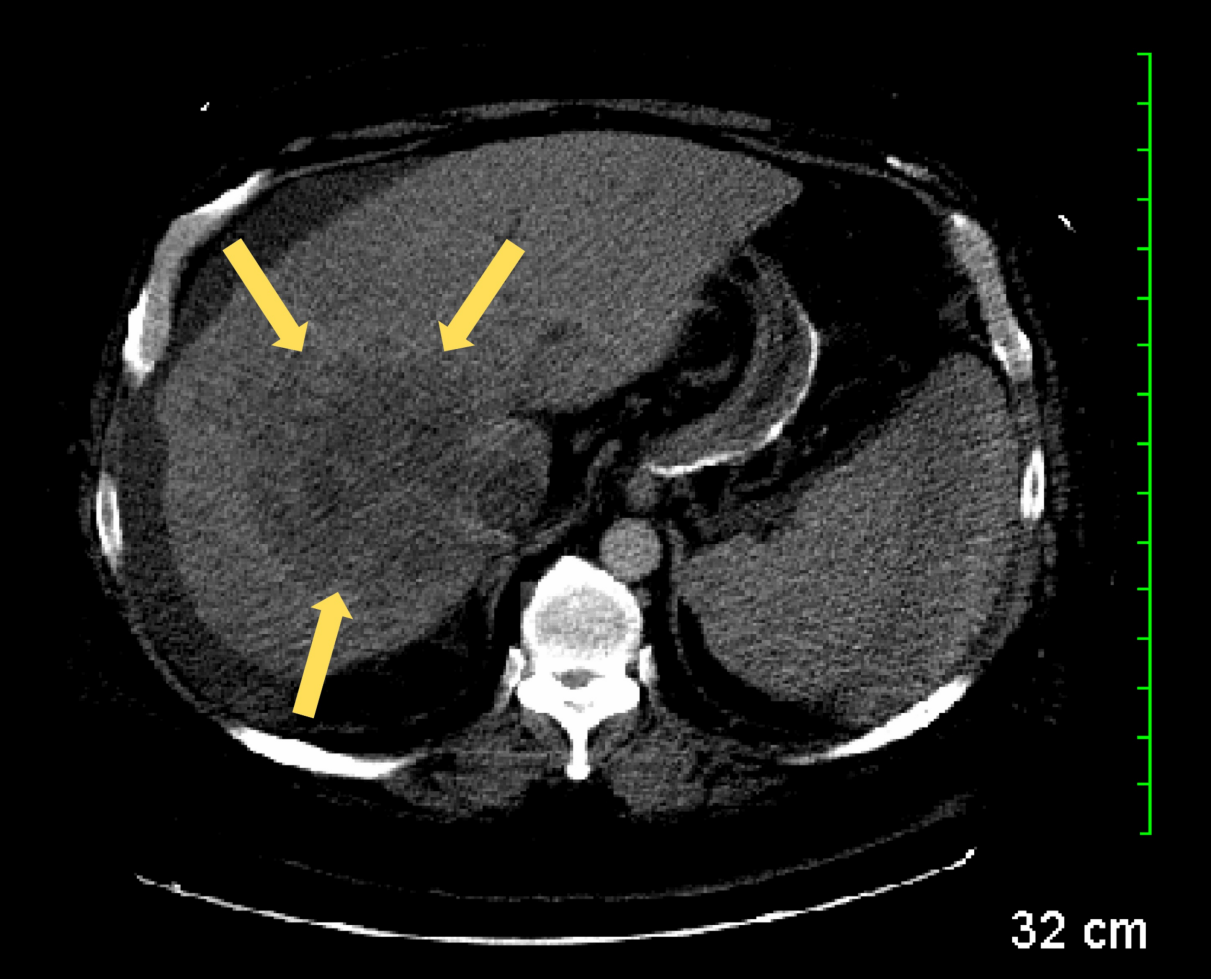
CT scan revealing multifocal masses (yellow arrows) predominantly involving the right lobe of the liver. CT: computed tomography
